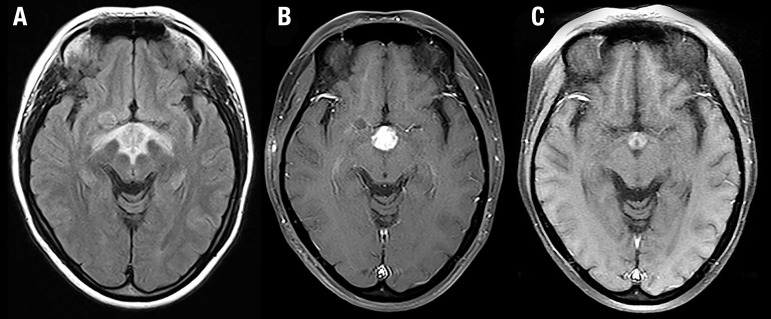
Case 2. Initial MRI. Axial FLAIR [A] and axial contrast-enhanced
